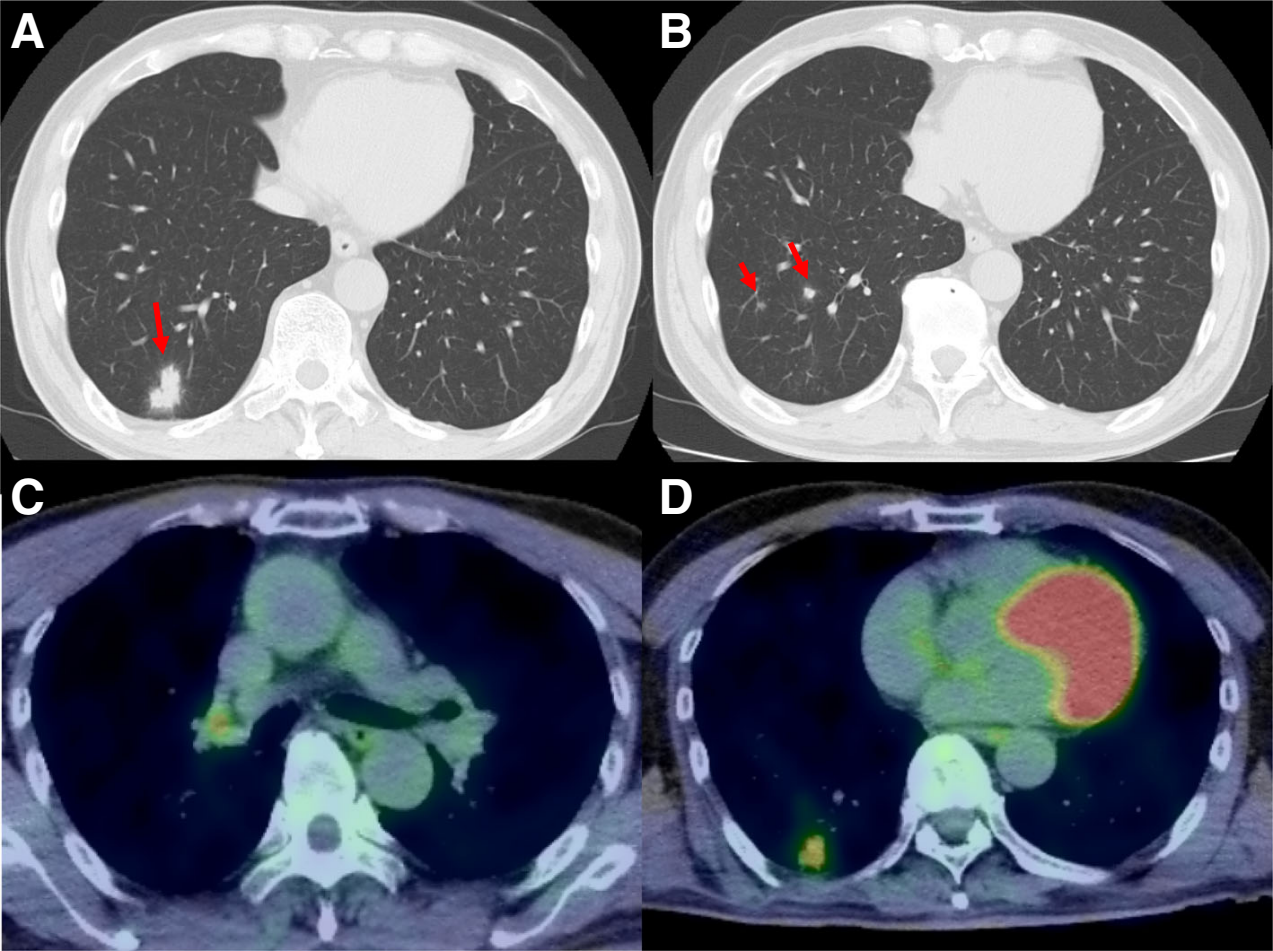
Imaging findings. a Computed tomography showing the pulmonary nodule (24 mm) in the posterior basal segment of the right lung. b Computed tomography showing two small nodules (8 mm and 6 mm) in the posterior basal segment of the right lung. c Fluorodeoxyglucose positron emission tomography showing abnormal uptake in the main nodule. d Fluorodeoxyglucose positron emission tomography showing abnormal uptake in right hilar lymph nodes
